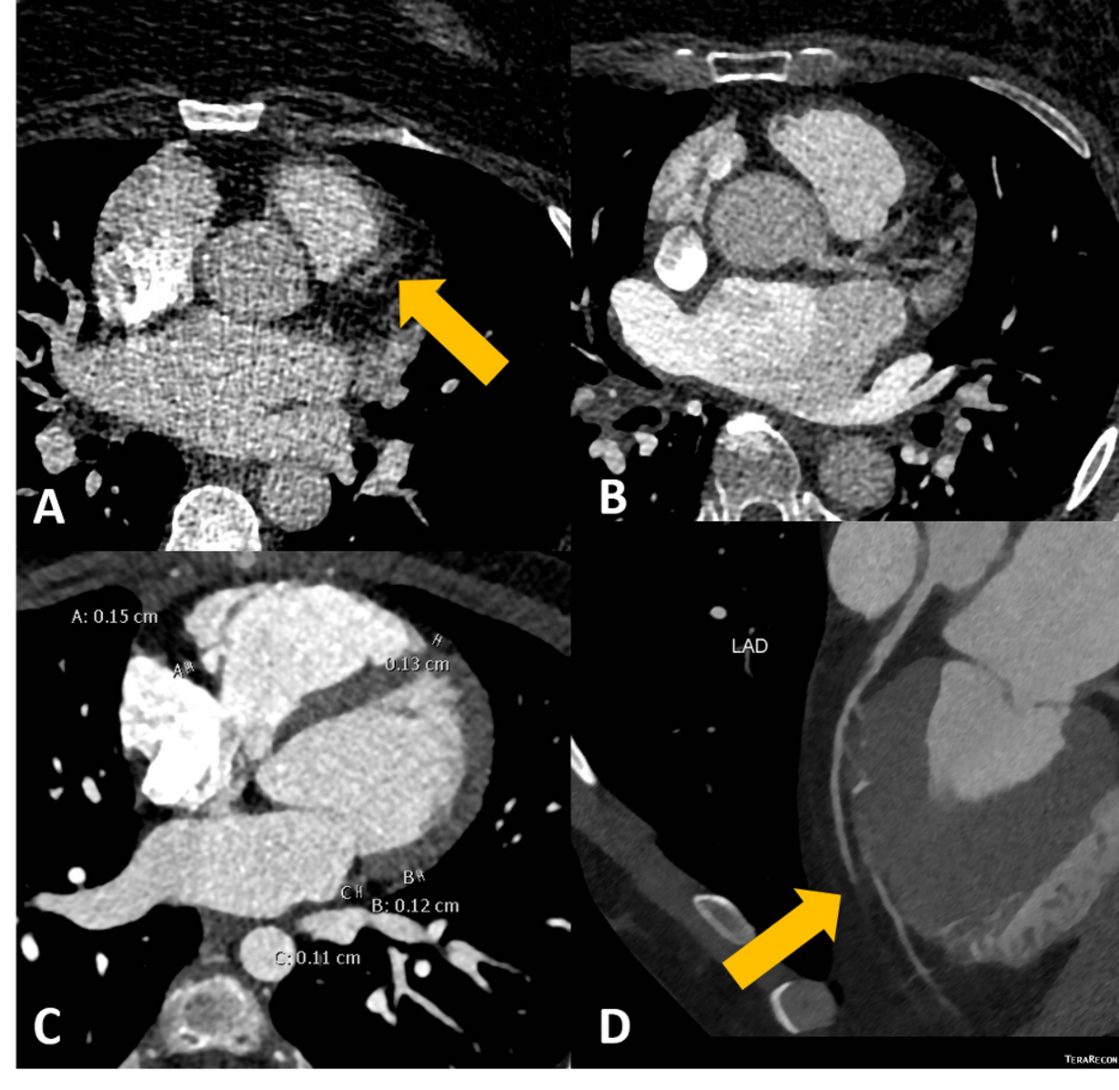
CCTA images demonstrating cardiac motion, respiratory motion, and poor opacification of coronary arteries. CCTA images demonstrating (A) extensive cardiac motion secondary to a high heart rate of greater than 70 beats per minute, poor contrast bolus timing, and quantum mottling artifact, resulting in poor visualization of the LAD (yellow arrow). (B) Poor contrast timing with the bolus of contrast predominately visualized in the left atrium. (C) Small coronary arteries without the administration of nitroglycerine. (D) Virtual reformat imaging demonstrating respiratory motion and an apparent break in the LAD (yellow arrow). All of these imaging factors limit the appropriate assessment of the coronary arteries. These examinations were subsequently rejected for FFRCT analysis. CCTA, coronary CT angiography; FFRCT, fractional flow reserve derived from CT angiography; LAD, left anterior descending artery
